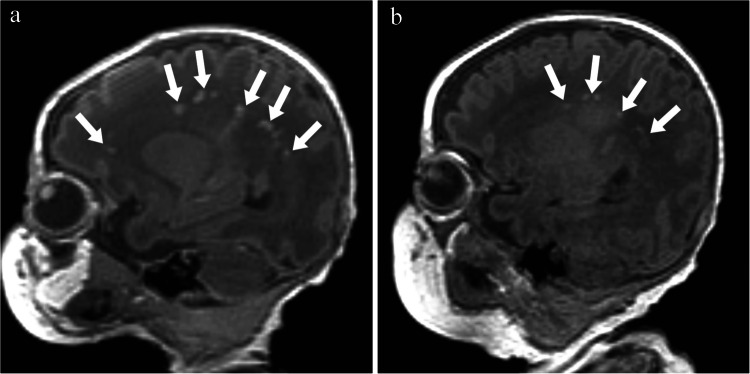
Parasagittal T1 magnetic resonance images without contrast of a boy born at 32 + 6 weeks. a The image obtained on day 9 (34 + 1 weeks) shows multiple areas of hyperintensity involving > 5% of the hemisphere, indicating severe white matter injury (*arrows*). b The image obtained on day 49 at 39 + 6 weeks shows decreased intensity and size of most lesions, with apparent resolution of some (*arrows*)
